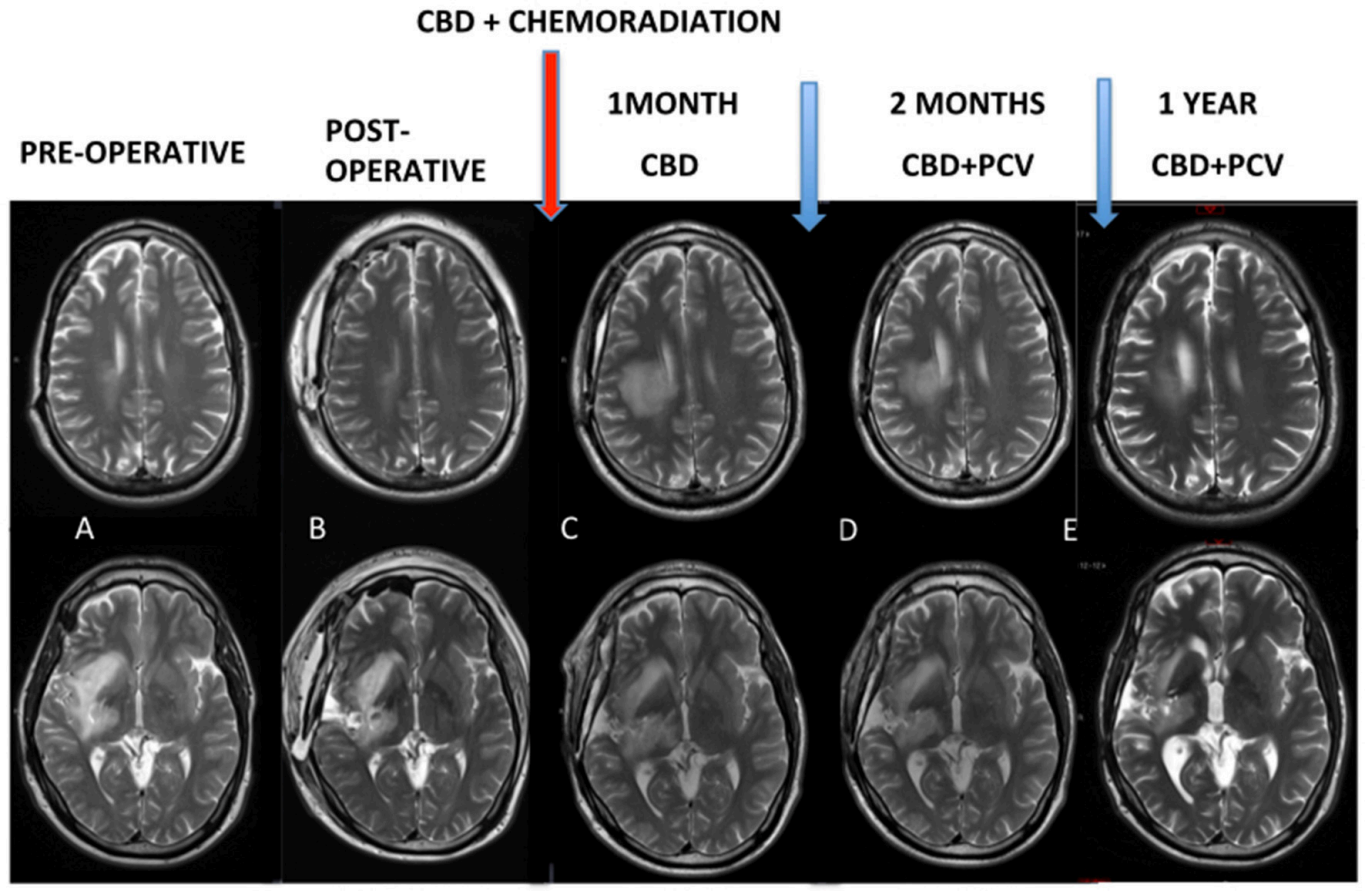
(A,B) Pre and post-operative MRI. Red arrow: chemoradiation associated with cannabidiol in a dosage ranging from 300 to 450 mg/day. (C) Control MRI after 1 month of the end of the chemoradiation was characterized an exacerbated and ultra-precocious phenomenon of pseudoprogression with increased edema and inflammatory disease. (D,E) MRI controls demonstrated progressive reduction of these findings.
